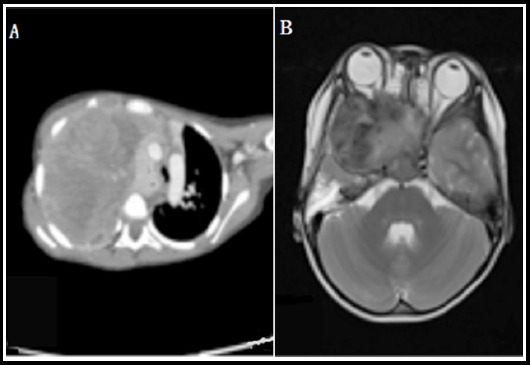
Imaging data of children with RMS at different sites. A: Thoracic CT, B: Cranial MRI.
